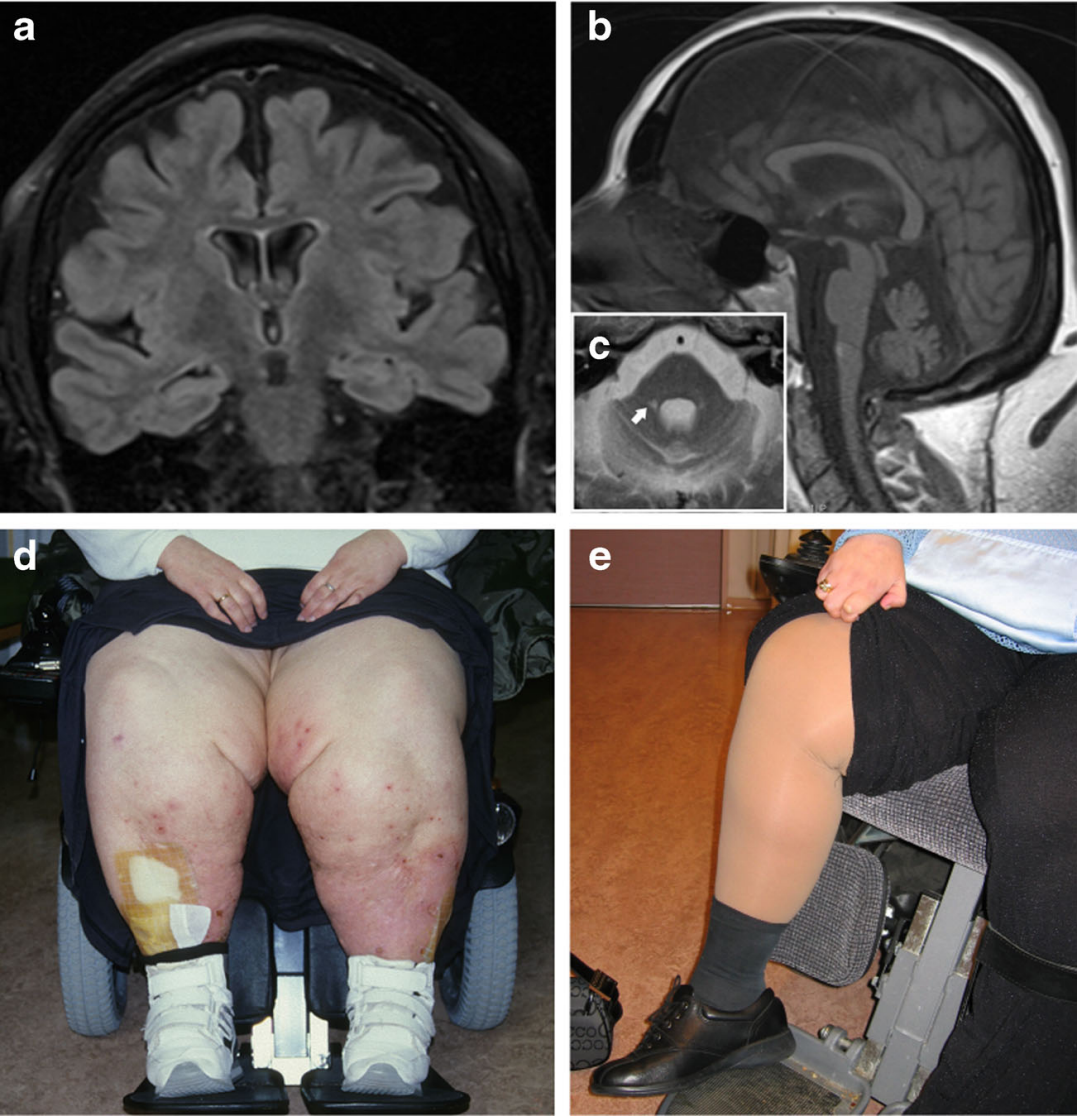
Clinical and radiological features of our patient. a Coronal FLAIR-T2 MRI showing generalized cerebral atrophy and ex vacuo ventricular dilation. b Sagittal T1-weighted MRI showing midline cerebellar atrophy. c Axial T2-weighted MRI showing cerebellar and pontine atrophy with dilation of the fourth ventricle and punctate bilateral high signal lesions at the base of the middle cerebellar peduncles (*arrow*). d Photograph of the patient showing severe bilateral edema of the lower limbs. e Improvement of the edema after albumin infusion and compression treatment
